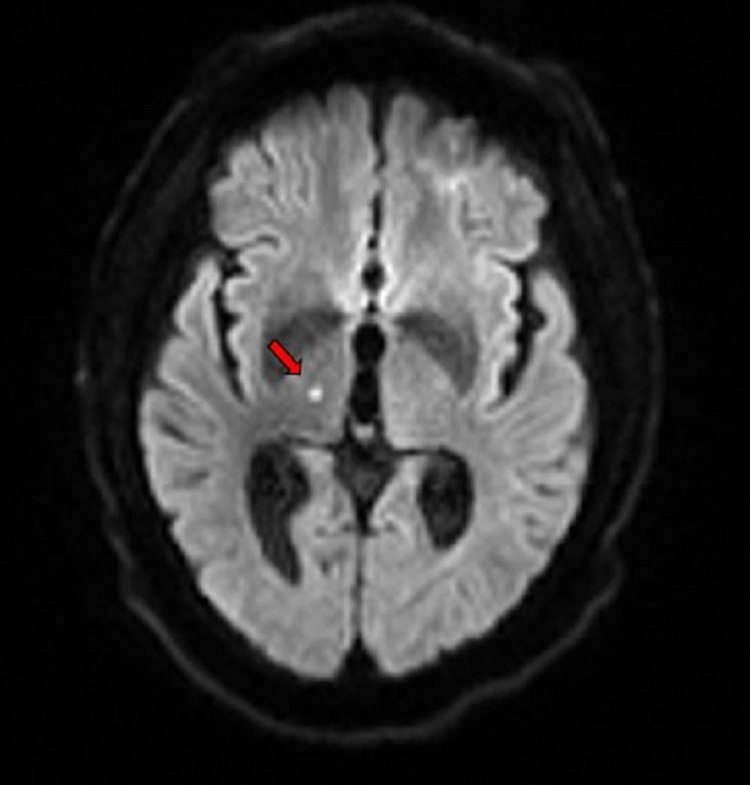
MRI of the brain showing punctuate foci of restricted diffusion in the right thalamus (red arrow) consistent with an acute lacunar infarct.
